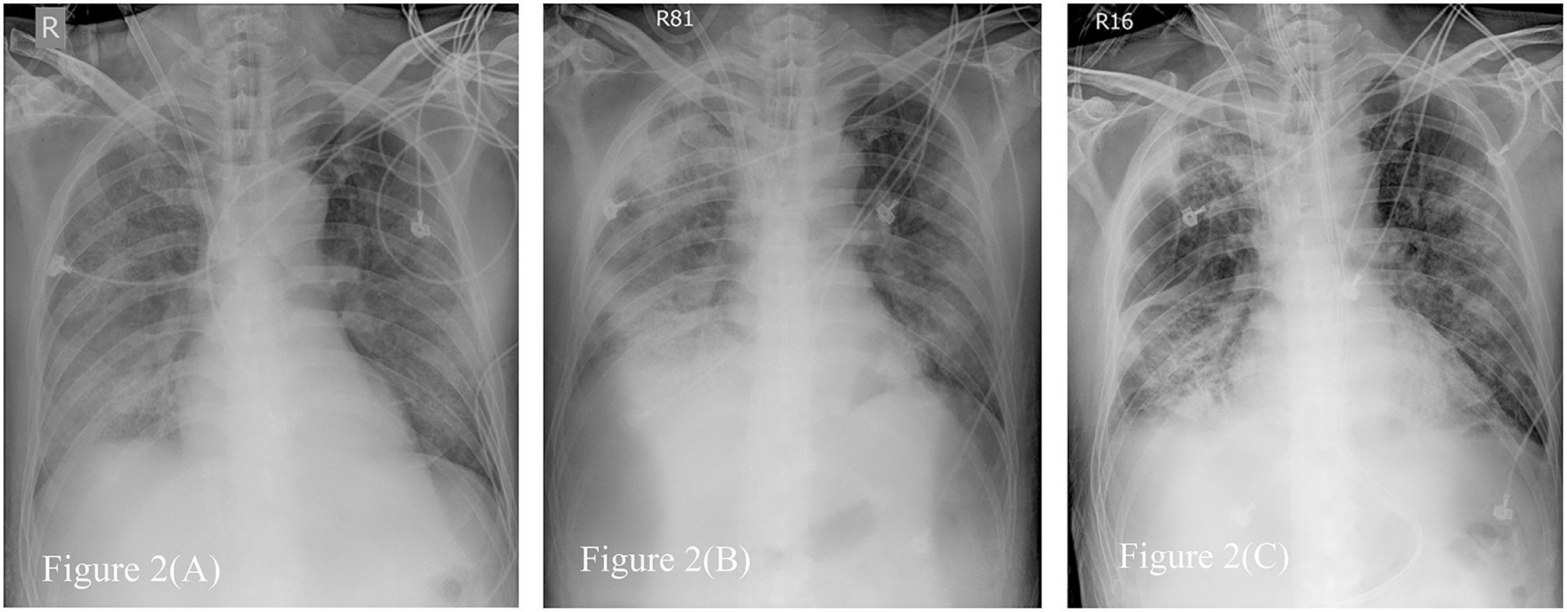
Serial Chest radiographs of the patient. (A) The film showing diffuse alveolar process over bilateral lung field, especially right lower lung field just after extracorporeal membrane oxygenator (ECMO setup). (B) The film revealing consolidation over both lungs when first time we tried to wean from ECMO. (C) The film on the day before removing ECMO, demonstrating fibrotic change as lung filed in (B).
